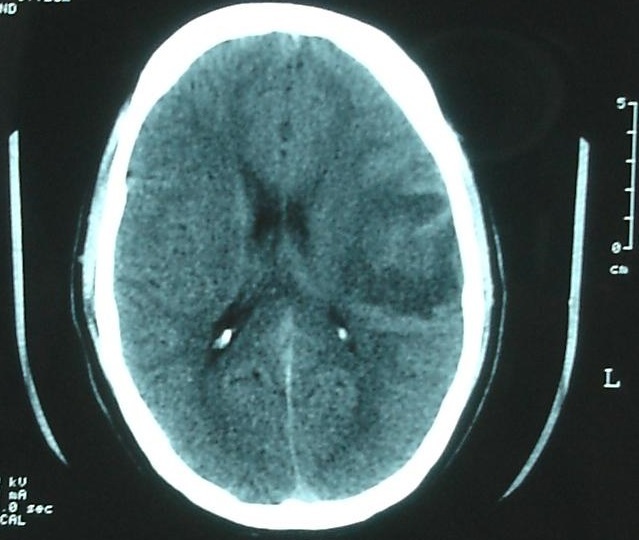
CT image showing a heterogeneous mass with surrounding brain edema in the left temporal region
